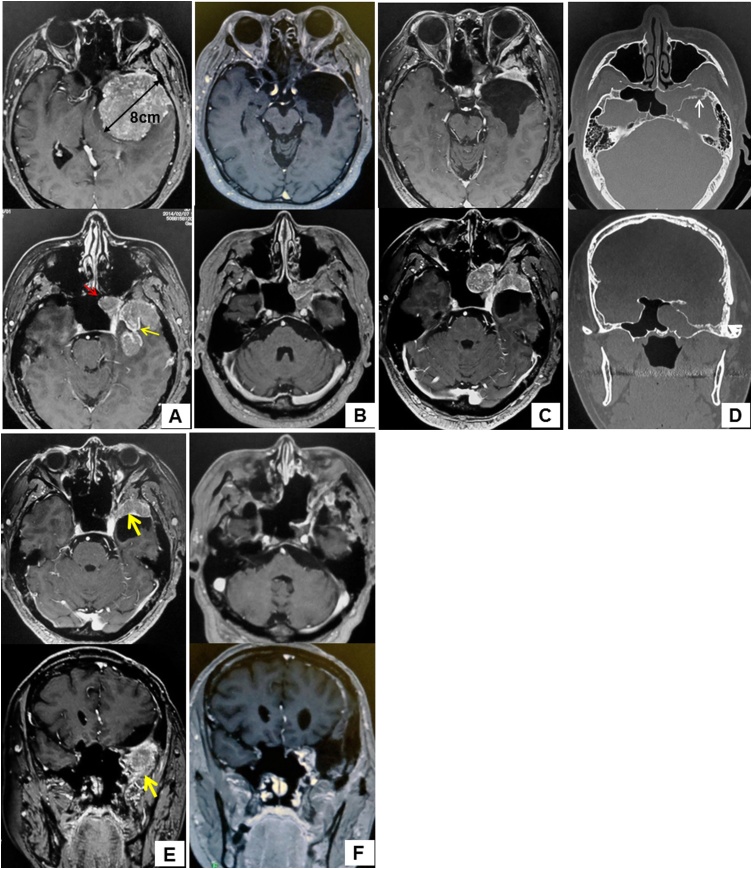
(A) A magnetic resonance image with gadolinium enhancement obtained at the initial visit shows an ovoid tumor with a maximum diameter of 8 cm on the left sphenoid ridge. The tumor was exerting strong pressure on the brain and was progressing further from the tip of the temporal bone (yellow arrow) into the sphenoid sinus (red arrow). (B) A magnetic resonance image with gadolinium enhancement obtained after a subtotal tumor excision by craniotomy. The temporal lobe tumor was removed but the tumor at the tip of the temporal bone to the sphenoid sinus remained. (C) A magnetic resonance image with gadolinium enhancement obtained 20 months after the subtotal tumor excision. The tumor remaining at the tip of the temporal bone to the sphenoid sinus was increasing in size. (D) A preoperative non-contrast-enhanced computed tomography image obtained before surgery via the endoscopic endonasal approach shows that the lateral recess of the sphenoid sinus was markedly enlarged and there was a soft tissue shadow occupying the left sphenoid sinus. Erosion was confirmed in the part of the bone bordering the lateral tip of the temporal lobe, suggesting a bone fracture in the order of several millimeters at this site (white arrow). (E) A magnetic resonance image with gadolinium enhancement obtained after surgery via the endoscopic endonasal approach confirming that the tumor within the sphenoid sinus had been completely removed, with residual tumor tissue remaining only at the tip of the left temporal lobe (yellow arrow). (F) A magnetic resonance image with gadolinium enhancement obtained after total resection of the residual tumor via craniotomy. No presence or recurrence of the tumor within the cranium or sphenoid sinus was observed.
